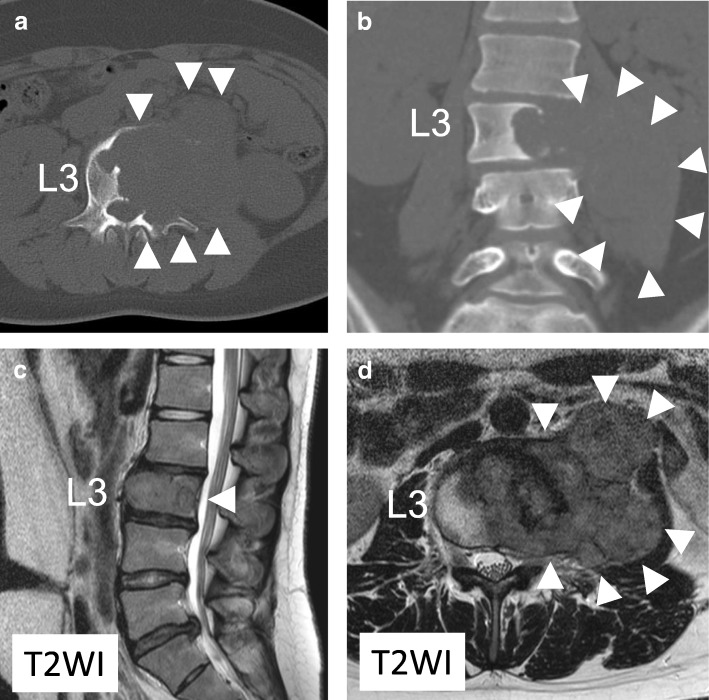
a, b Computed tomography prior to denosumab treatment, showing an osteolytic lesion involving the L3 vertebral body, which collapsed and expanded into the left side of the iliopsoas muscle (*arrowhead*). c, d T2-weighted magnetic resonance images prior to denosumab therapy, showing the tumor extending toward the left side of the paravertebral soft tissue (*arrowhead*). *T2WI* T2-weighted image
